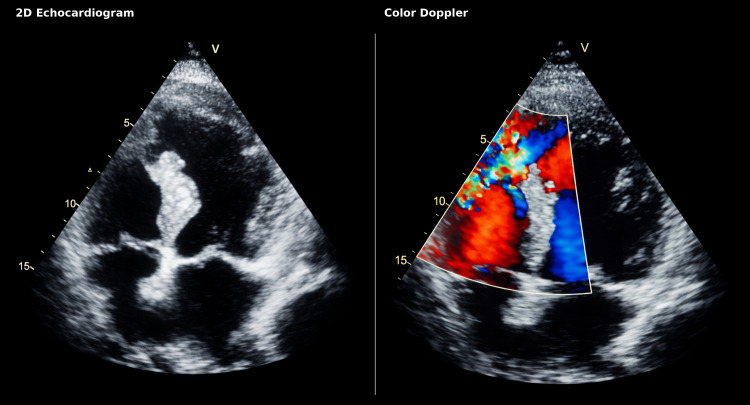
Apical four-chamber echocardiographic view The images demonstrate inferoseptal wall thinning and post-infarction ventricular septal rupture. Apical four-chamber transthoracic echocardiographic view showing thinning of the apical inferoseptal septum with an irregular “bite-like” appearance secondary to septal rupture on two-dimensional imaging (left panel). Color Doppler (right panel) demonstrates a left-to-right shunt across the interventricular septum, confirming post-infarction ventricular septal rupture.
